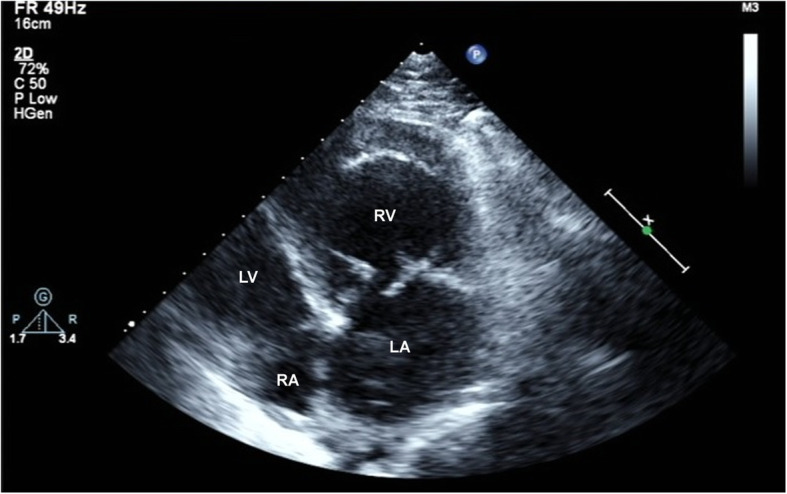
Apical four-chamber view showed the valve in morphological left ventricle was inferior to the valve in the right ventricle. Anterior leaflet of tricuspid valve receded to left atrium and left a large gap with other leaflets in systole period. LA = left atrial; RA = right atrial; RV = right ventricle; LV = left ventricle
